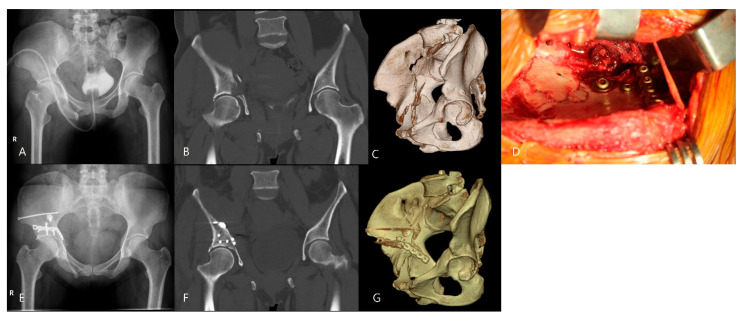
Standard anteroposterior radiograph (A) of a fracture of the right acetabulum. Coronal reconstruction CT scan (B) and three-dimensional CT scan (C) showing an incomplete anterior column fracture with a displaced quadrilateral plate fracture. Intraoperative photograph (D) showing anatomical reduction in the fracture and fixation with a lag screw and a spring plate. Postoperative anteroposterior radiograph (E) of the anterior column fracture stabilized using a supraacetabular lag screw and a spring plate. Postoperative sagittal reconstruction (F) and three-dimensional CT scan (G) showing anatomical reduction at the weight-bearing surface.
